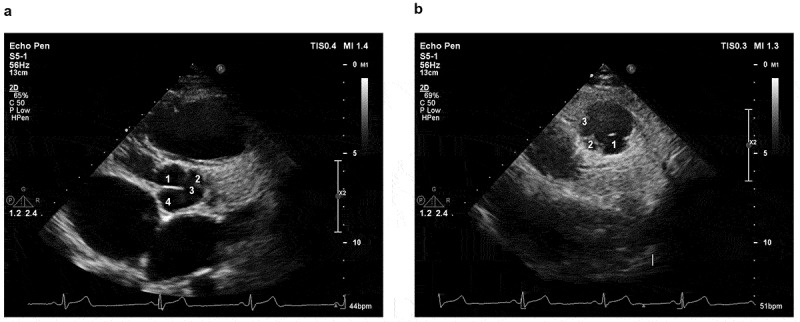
Echocardiographic image of the patient with combined malformation of aortic valve and pulmonary valve. (a). Short-axis transesophageal echocardiogram (TEE) image shows four cusps of the aortic valve. (b). Short-axis TEE image shows more than three cusps of the pulmonary valve.
